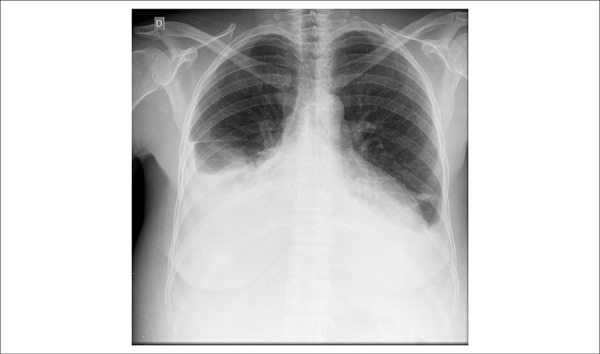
Chest X-ray showing bilateral veiling of costophrenic sinus, with pleural
